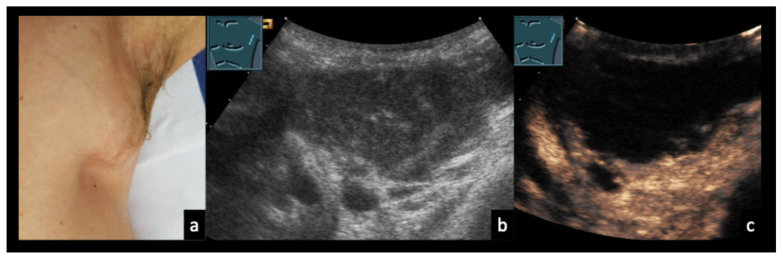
34-year-old male patient (a) with confirmed axillary lymph node tularemia (*Francisella tularensis*). (b) B-mode ultrasound shows heterogeneous, confluent lymph node formations. (c) On CEUS at 40 s, there is near-complete absence of enhancement, consistent with abscess formation.
